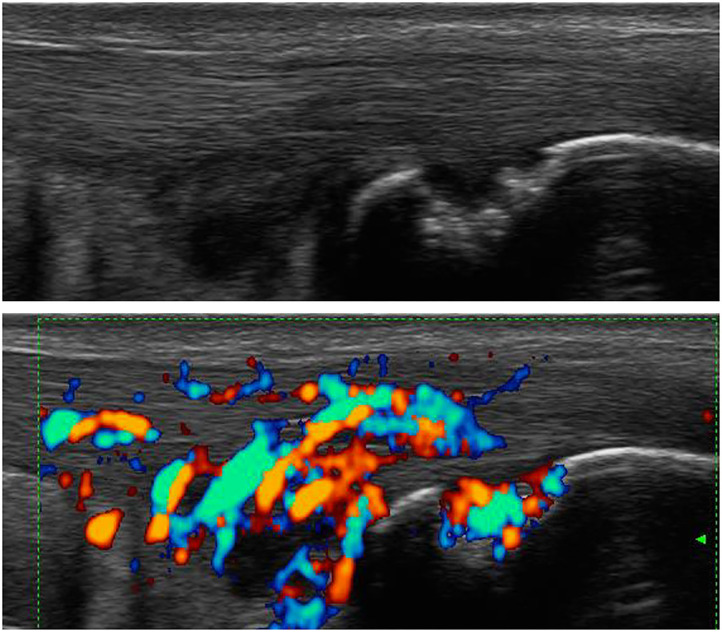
Ultrasound assessment. Tendinitis of the Achilles tendon with the formation of osteophytes and power Doppler activity.
